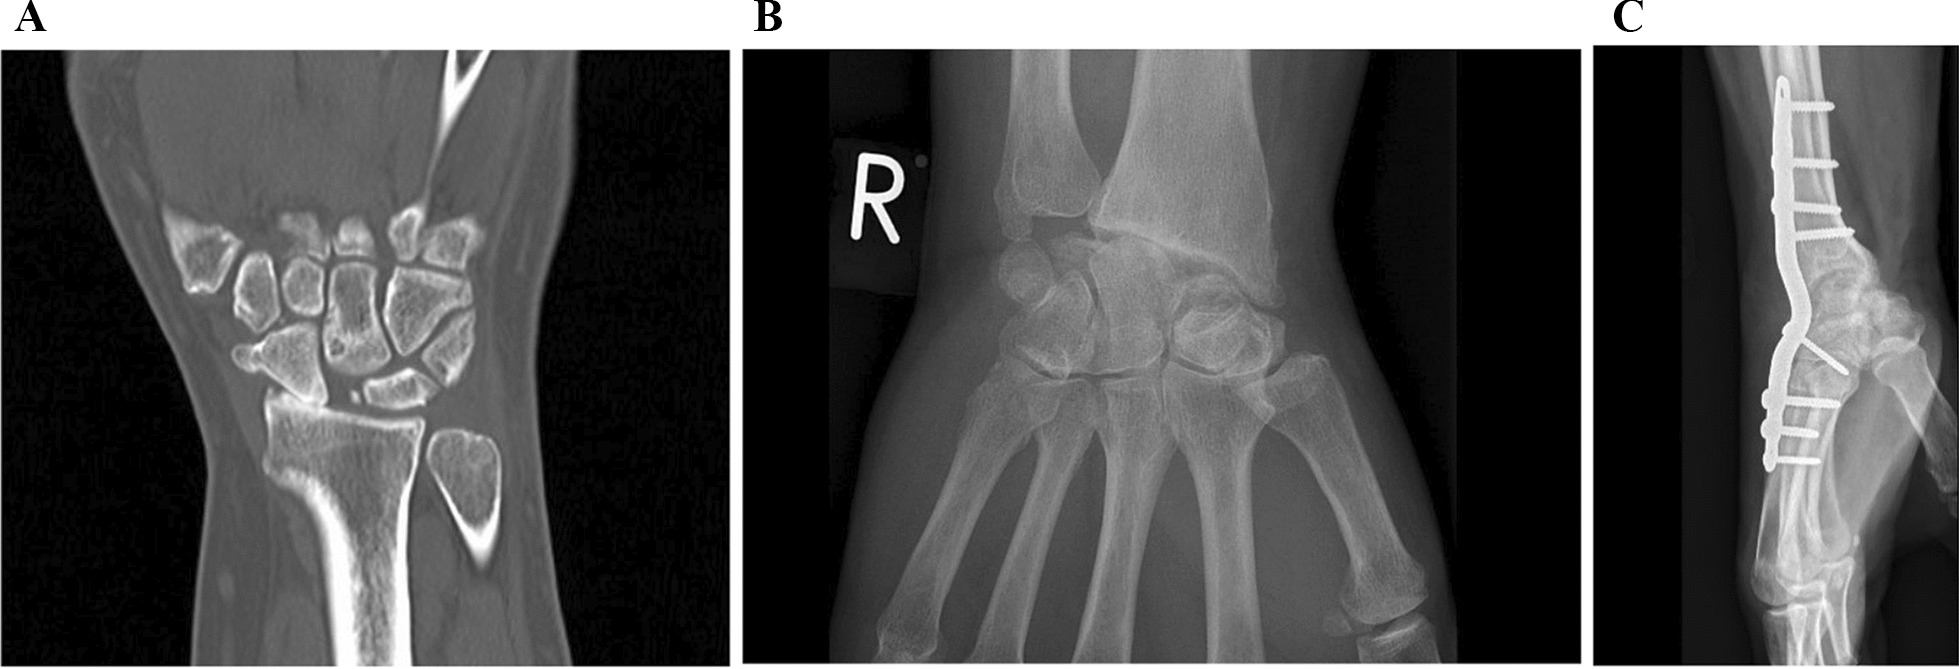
A 57-year-old male with a SLAC wrist. Preoperative CT scan of the right wrist (coronal plane) showing lunate fossa of the radius, free of radiological degenerative changes, justifying the PRC procedure. B Postoperative X-ray (P-A plan) showing clear signs of osteoarthritis, 14 months after the primary PRC procedure. C X-ray (side plan) of the patient’s wrist, 21 months after the primary procedure. The wrist has been fused due to severe pain
